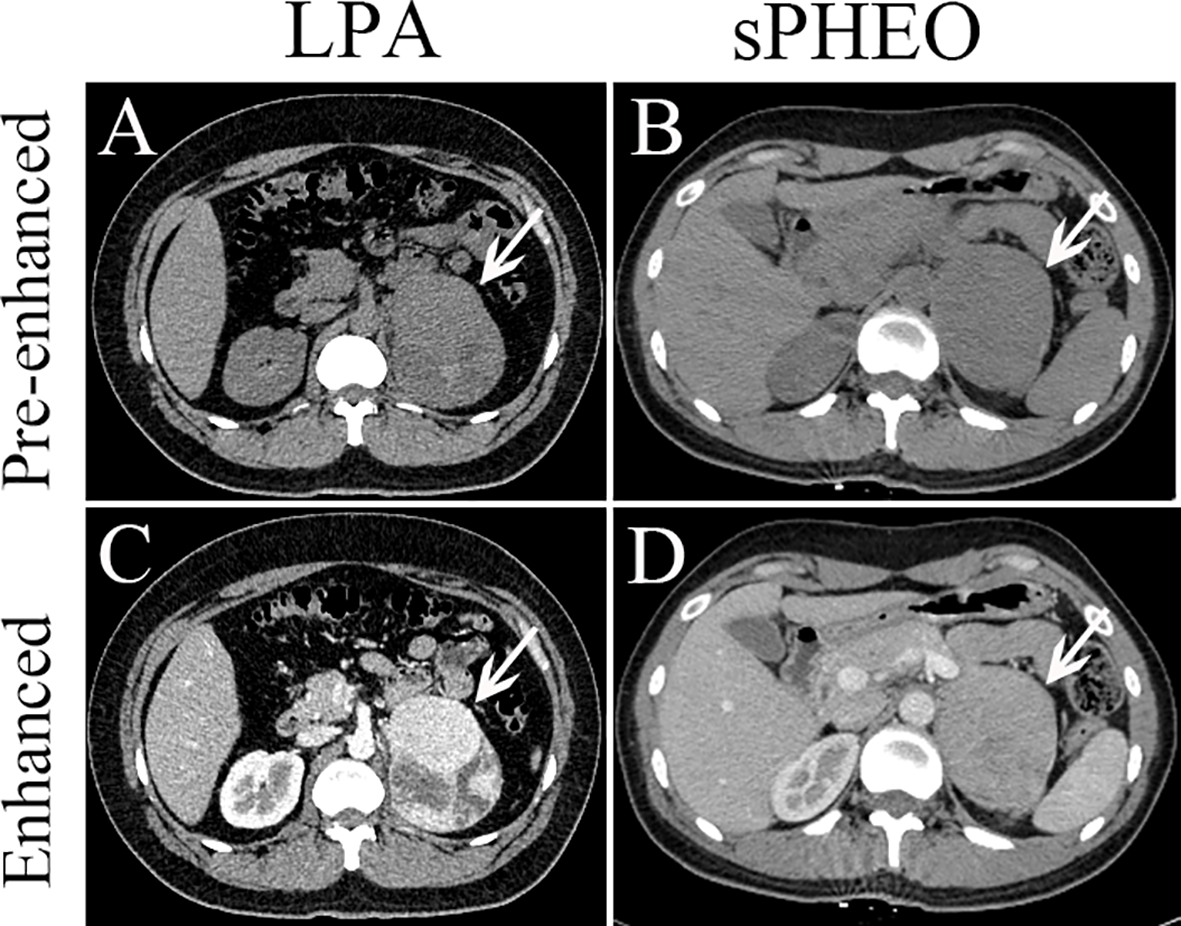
Axial pre-enhanced and enhanced CT images of a patient with lipid-poor adenomas (LPA) (Case 95) and a patient with subclinical pheochromocytoma (sPHEO) (Case 21) showing left adrenal mass at the tumor largest dimensions. On pre-enhanced images, the LPA appeared as an irregular mass with intermediate heterogeneous density (A), while the sPHEO was an elliptical mass with relatively homogeneous density (B). After injection of contrast medium (65s), the LPA was markedly more heterogeneous, with obvious cystic and necrotic areas (C), while the sPHEO showed a mildly heterogeneous enhancement pattern (D).
